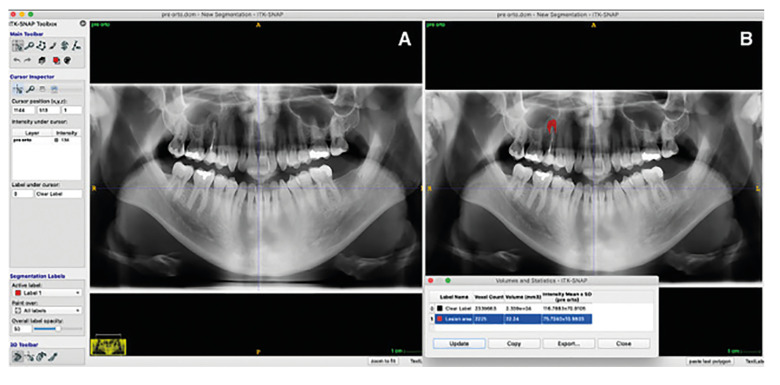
a. Panoramic radiography showing the periapical area in the same 2nd premolar. b. Measurement performed manually in the program Itk-Snap (22.24 mm2).
